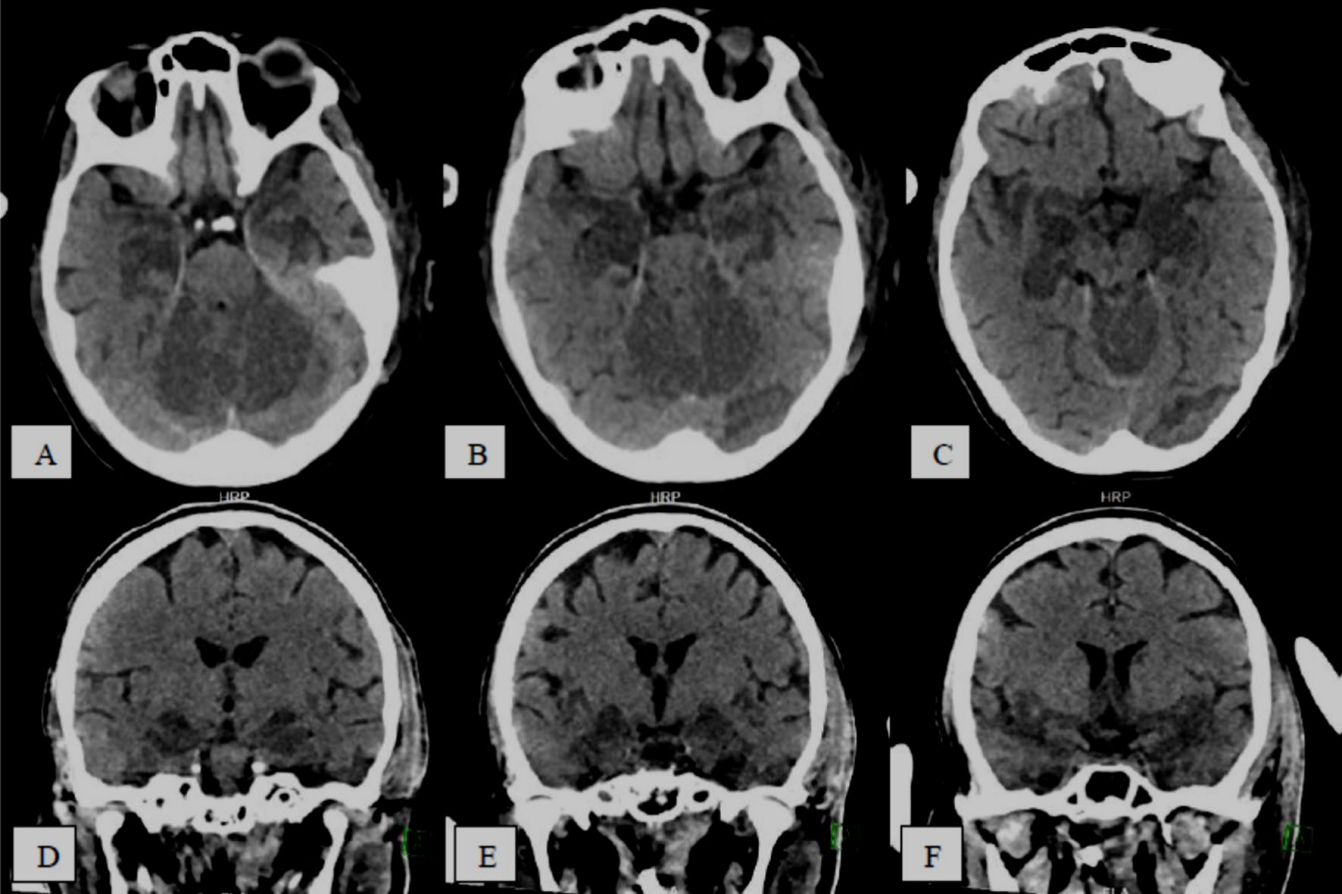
22-year-old-female COVID-19 positive patient complaining of worsening pneumonia and difficult to oxygenate. Presented with impaired level of consciousness, A–C and D–F serial axial and coronal CT images, respectively, show bilateral cerebellar and medial temporal lobe hypo-attenuation with relative loss of gray white matter differentiation, suggestive of *hypoxic*
